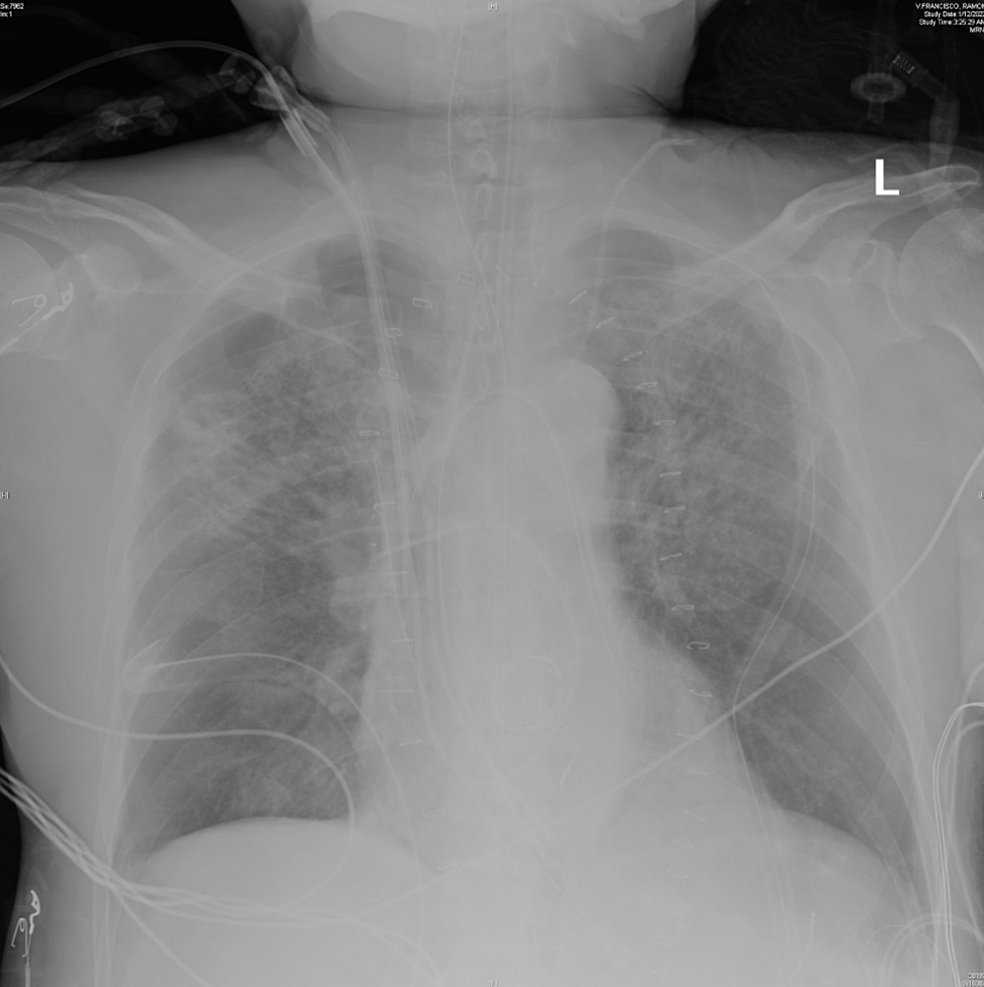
Chest x-ray (CXR) taken in the ICU postoperatively with an open and packed sternotomy demonstrating multifocal pneumonia with appropriately placed lines and drains.
